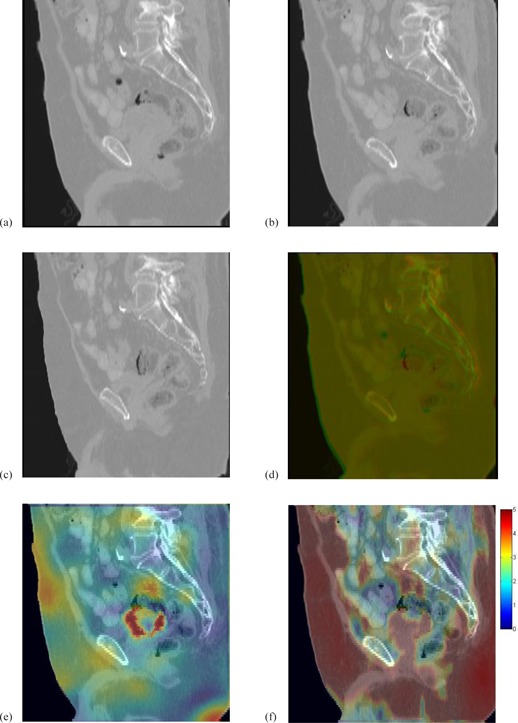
The FEM constructed image $P_{1}^{\text{CT}}$ (a); the prostate CT images (b) and (c) warped by DMP‐DVF and Demons‐DVF; (d) overlay of the warped prostate CT images in (a) and (b); (e) and (f) are the displacement errors (mm) of the DMP registration and the Demons registration overlaid on a sagittal view of the FEM constructed image $P_{1}^{\text{CT}}$.
